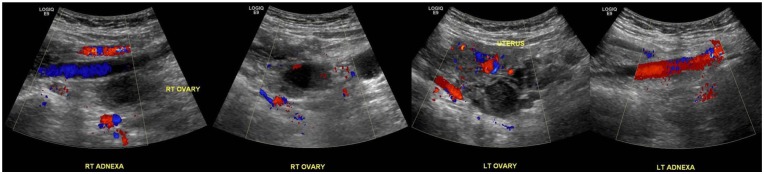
Ultrasound of pelvis did not show any obvious lesions or cystic
